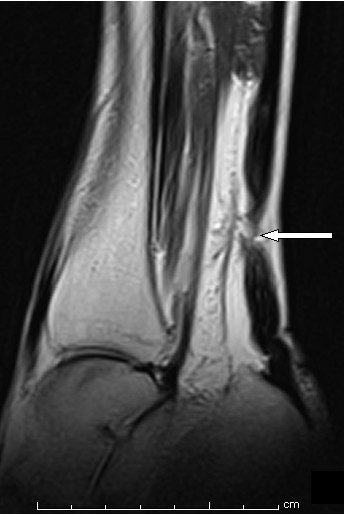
Sagittal view magnetic resonance imaging (MRI) scans of the patient's right ankle showing rupture of the Achilles tendon.
